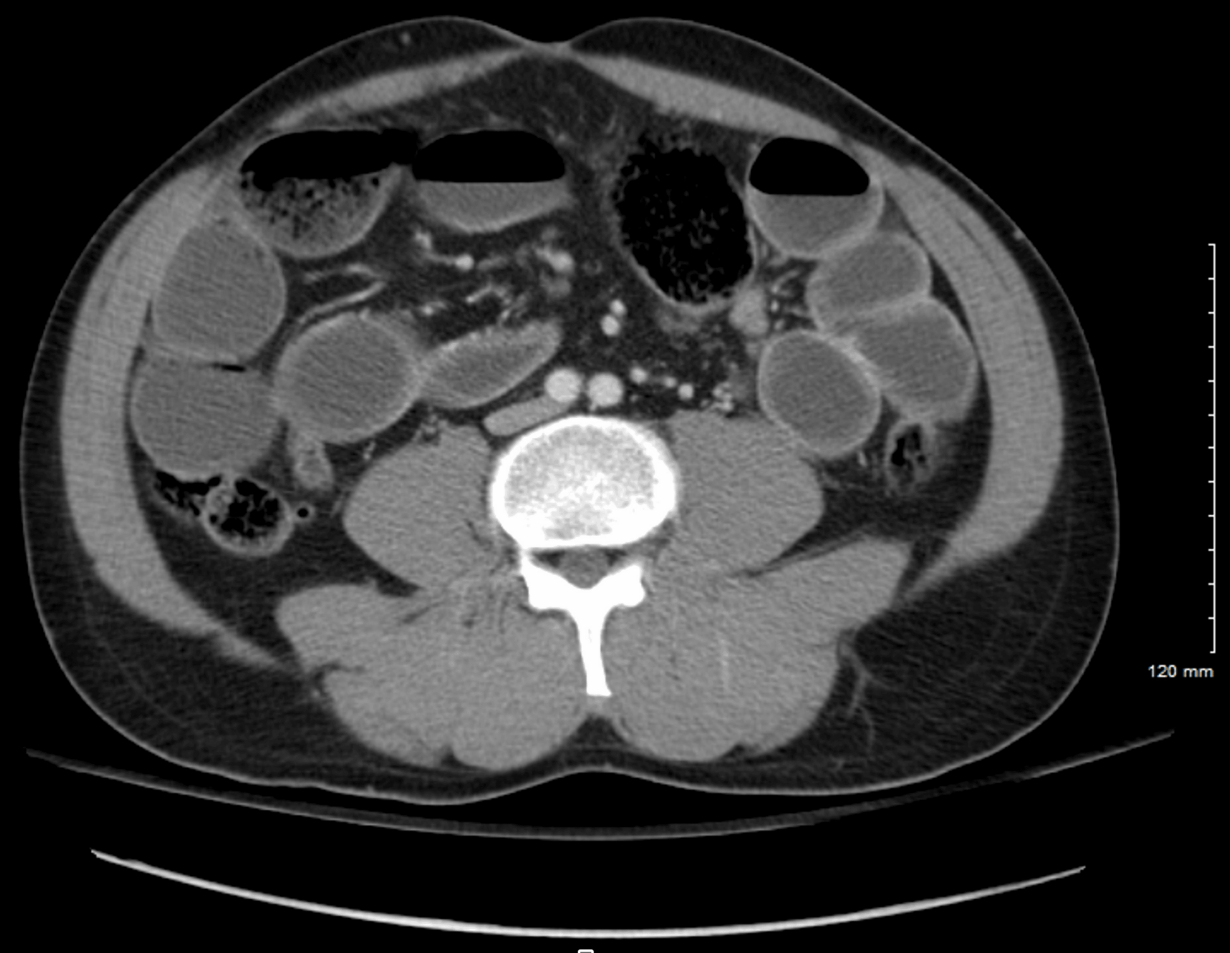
Case three: a CT scan of the abdomen and pelvis showing a small bowel obstruction, with the transition point in the right hemiabdomen at the site of a previous surgical change. There is a nonspecific bowel gas pattern, with no evidence of pneumoperitoneum. Moderate fecal loading is also present.
